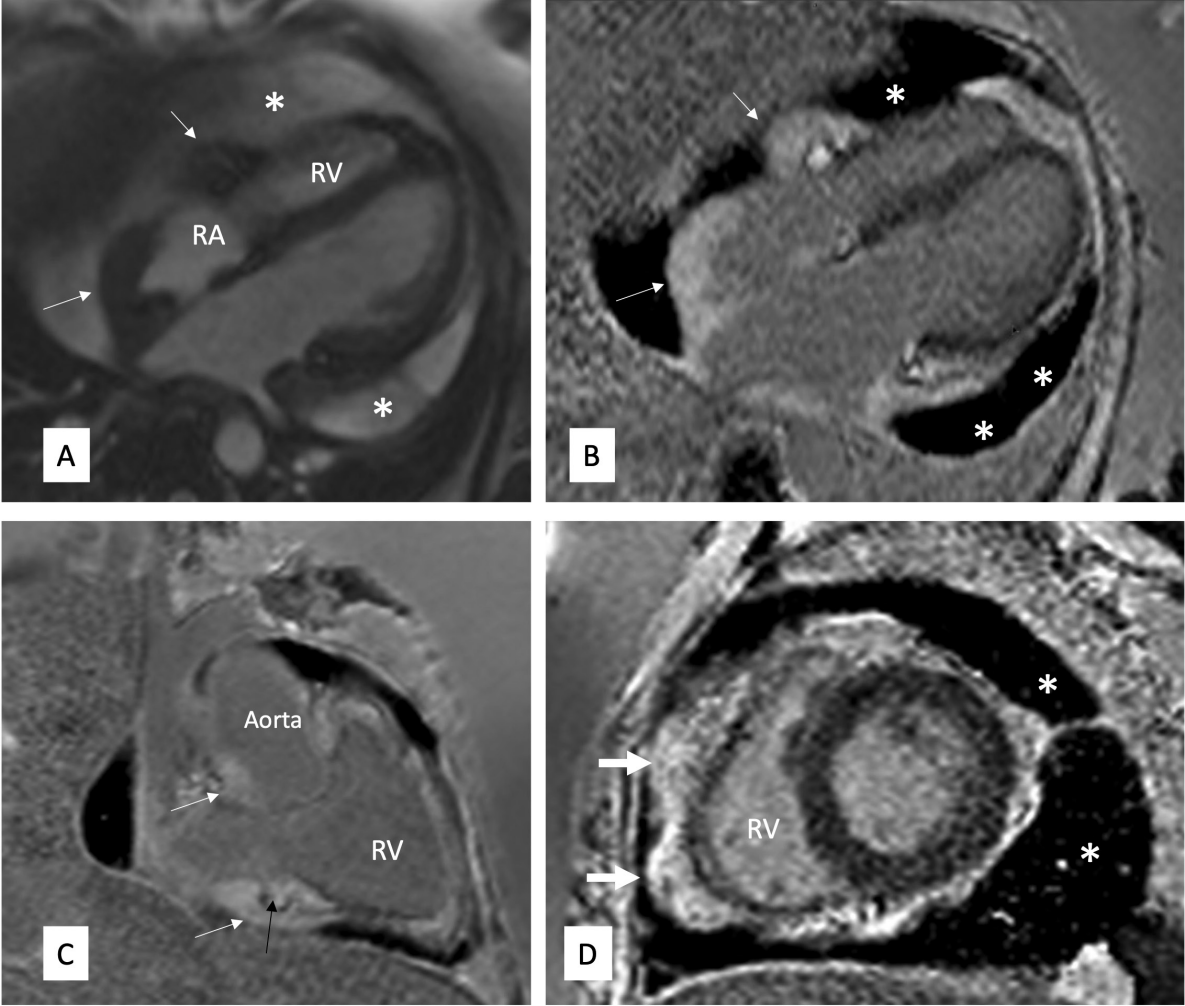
Cardiac MRI cine image in 4 chamber (A) view, late gadolinium enhancement images in 4 chamber (B), vertical long axis (C) and short axis (D) views show sheath-like thickening in the pericardium, right atrial (RA) wall, right atrioventricular groove encasing the right coronary artery (the black arrow in C), and also along the aortic root. This thickening also shows bright late gadolinium enhancement (B–D). Moderate pericardial effusion (white asterisks) is noted *Note*. RV: Right ventricle
